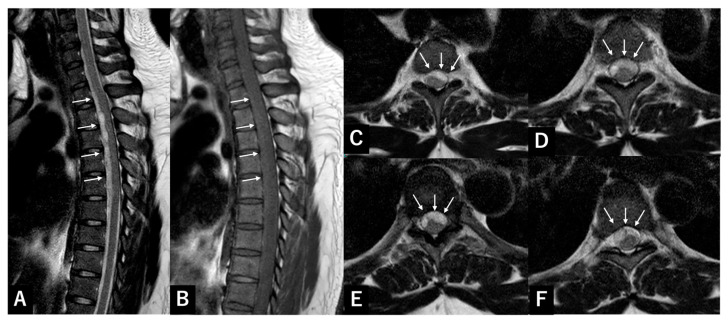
Representative case of SSSDH. (A) T2-weighted sagittal MRI of the cervicothoracic spine showing a ventral spinal hematoma (arrow) at T1–T6; (B) T1-weighted sagittal plane; (C) T2-weighted axial plane at T2/3; (D) T3/4; (E) T4/5; (F) T5/6. SSSDH: spontaneous spinal subdural hematoma; MRI: magnetic resonance imaging.
